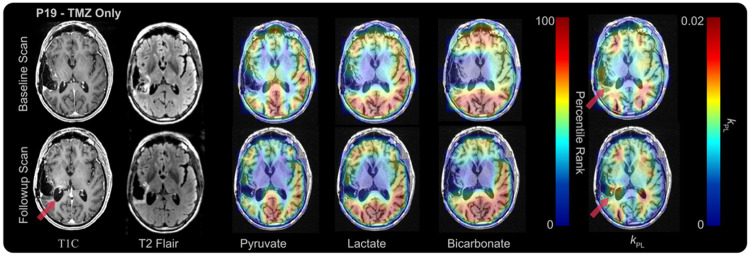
Representative slice for P19 at Pre-Tx (*top row*) and 1-month Post-Tx follow-up (*bottom row*). Post-contrast T1 and T2 FLAIR anatomical (panels 1-2), maps for Pyr%, Lac%, and Bic% (*panels 3-5*), and k_PL_ maps (*panel 6*) overlayed on the T1 image. Arrows indicate T2 lesion.
